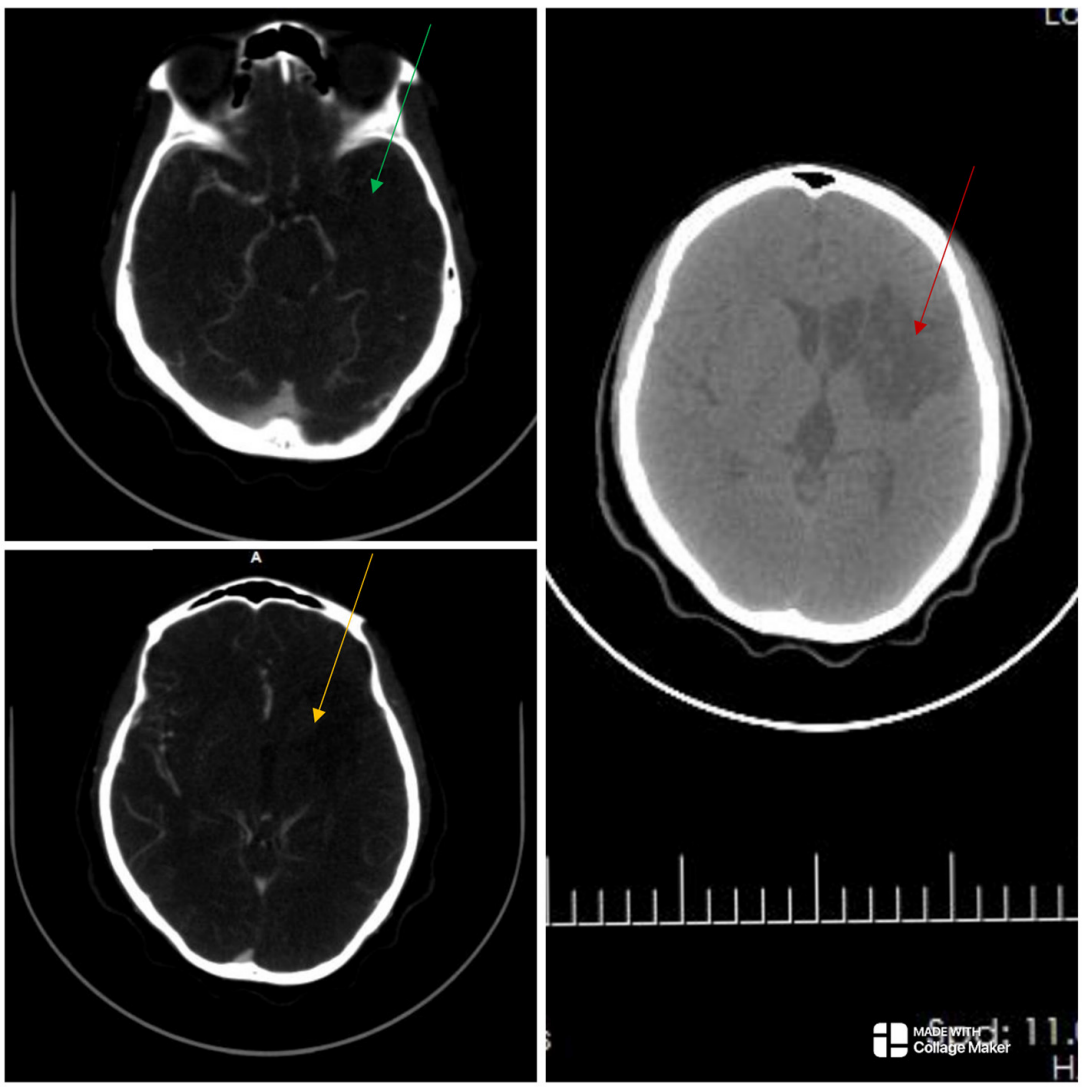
The cranial computed tomography (CT) scan (right) shows an ill-defined oval-shaped (red arrow), hypodense area (HU = 14–36) involving the left basal ganglia and frontal lobe in keeping with an acute infarct. The CT angiography (left) showed absent opacification at the origin of the right common carotid artery and a string-like lumen from C7/T1 to C4/5 (not shown). There was opacification at the origin of the left internal carotid artery (ICA) but the artery abruptly terminated just beyond that point. There was no opacification in the cervical or intracranial segments of the left ICA (green and yellow arrows), as well as in the left subclavian artery. There were short segment stenoses in the pulmonary artery branches bilaterally (not shown). Overall features showed diffuse vascular smooth muscle wall disease affecting both intracranial and extracranial vessels, in keeping with inflammatory vascular disease.
